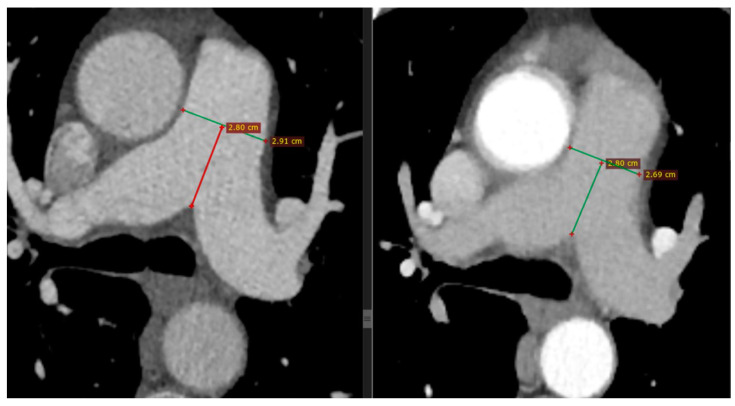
Representative chest CT images. Consecutive measurements of MPAD at mid-PA at the level of the mid-aortic arch and main carina on chest CT in a patient with COVID-19 pneumonia (left) and after 6 months (right), with a substantial decrease noted (from 2.91 to 2.69 cm). Horizontal green lines represent the MPAD, whereas vertical red and green lines represent the distance of MPAD from the bifurcation of the pulmonary artery.
